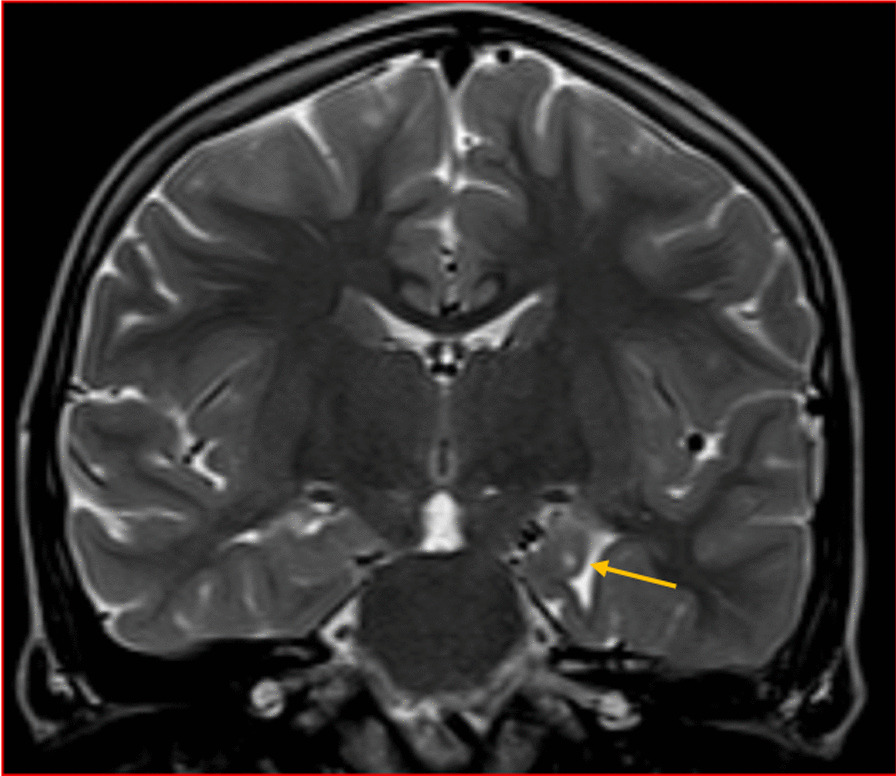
Six-year-old male with focal seizures. Coronal T2 weighted brain MRI demonstrating an abnormally shaped left hippocampal head (globular) with a vertically oriented collateral sulcus (arrow) in a case of incomplete left hippocampal inversion
